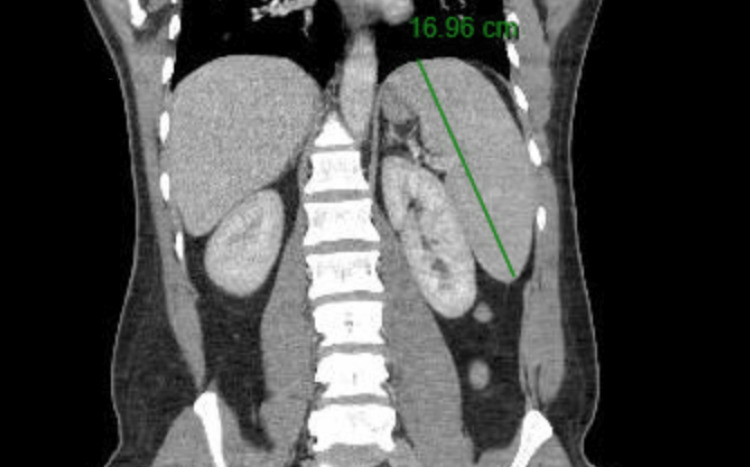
CT abdomen/pelvis with contrast showing splenomegaly (green line).
